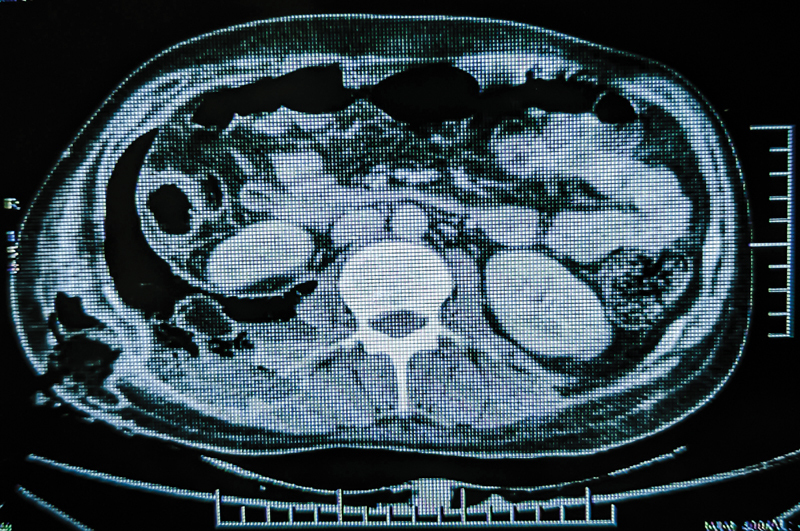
CECT abdomen showing pneumoretroperitoneum and hypodense collection on the right side. CECT, contrast-enhanced computed tomography.
